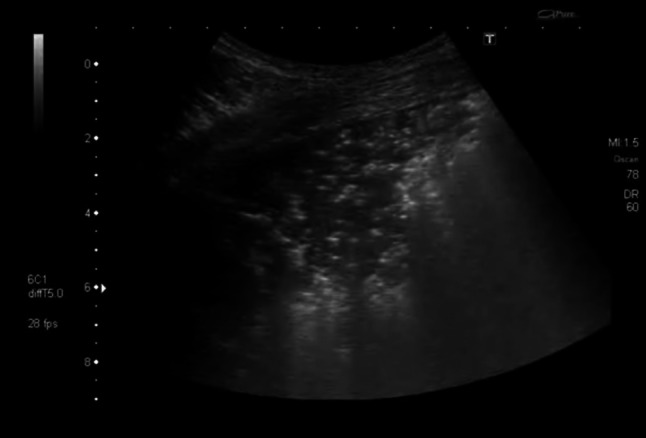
Transthoracic ultrasonography showing large consolidated areas with multiple bright artifacts suggesting gas inclusions. These represent both air bronchograms and cystic areas of the lung
